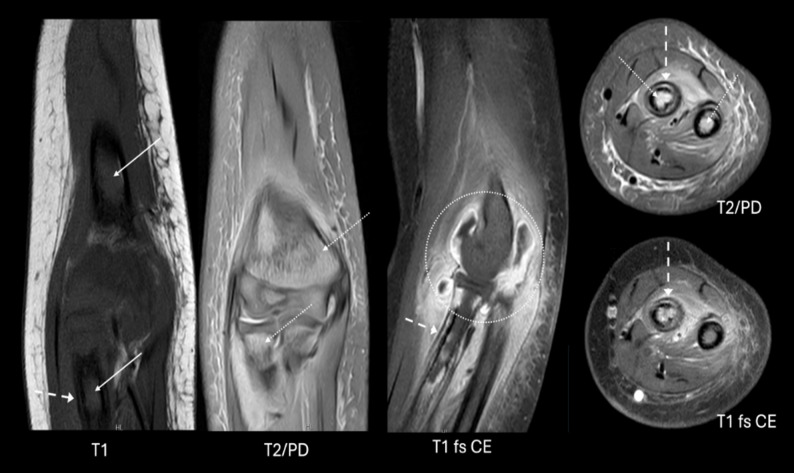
Patient 12. MRI of the right elbow shows bone marrow signal alterations characterized by T1w hypointensity (arrows) and T2w hyperintensity (dotted arrows). Joint effusion (dotted circle) and periosteal reaction (dashed arrow) are also indicated. This case is representative of the diagnostic challenge because inflammatory findings initially suggested osteomyelitis, whereas the diffuse marrow abnormalities ultimately prompted hematologic evaluation
